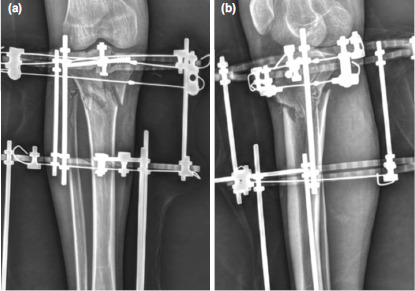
Case No.12, post-operative radiographs of the knee. (a) Antero-posterior view showing restoration of the condylar width, reduction of intra-articular step and stabilisation with an inter-fragmentary screw and counter acting olive wires using a ring fixator. (b) Lateral view showing well maintained posterior tibial slope.
